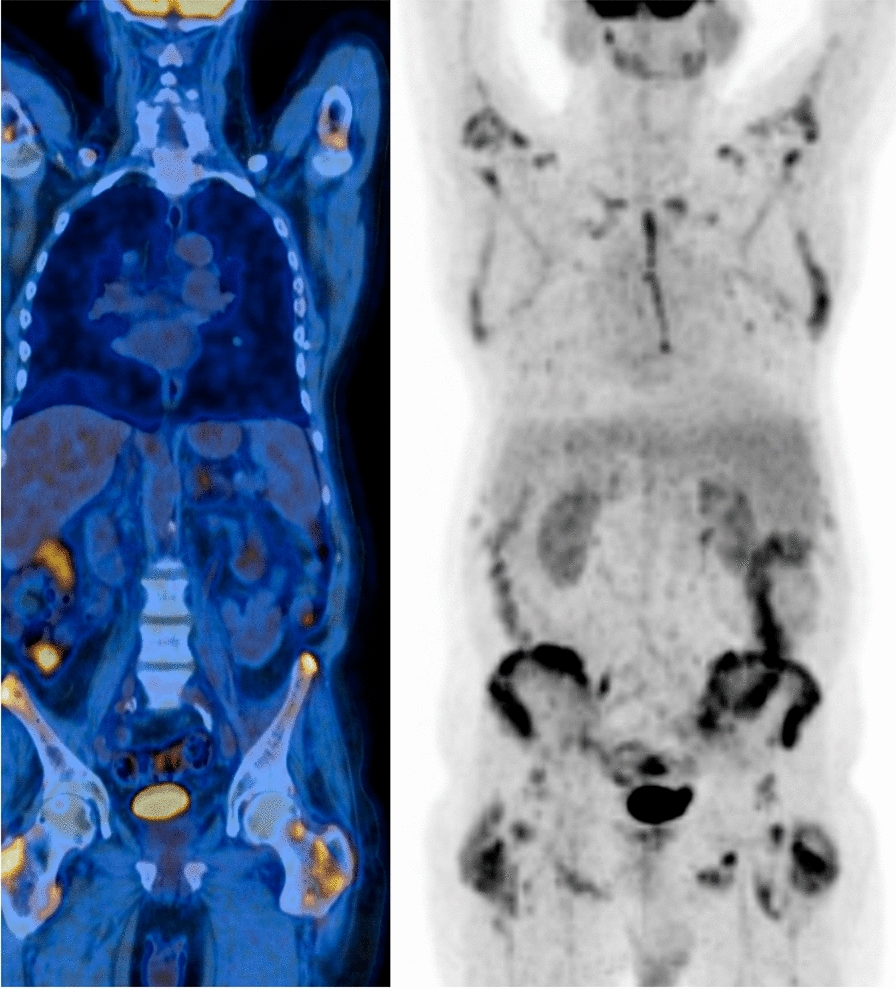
PET–CT and bone scan 1 month prior to admission showing tumor burden with pelvic bone lesions and metastatic lung disease
